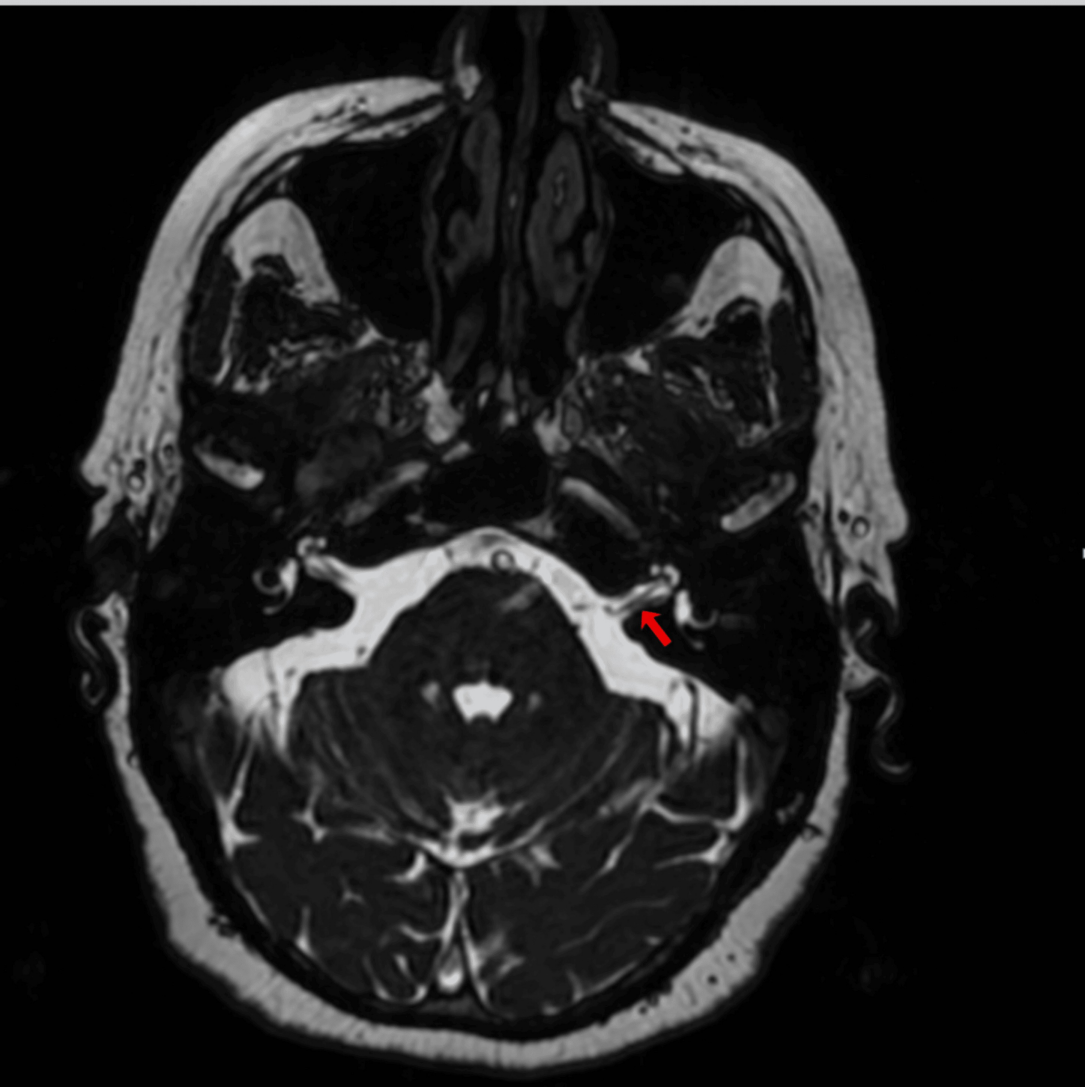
Axial FIESTA brain MRI of patient no.4 Red arrow: Left-sided neurovascular compression (Chavda classification grade III) FIESTA: Fast imaging employing steady-state acquisition
